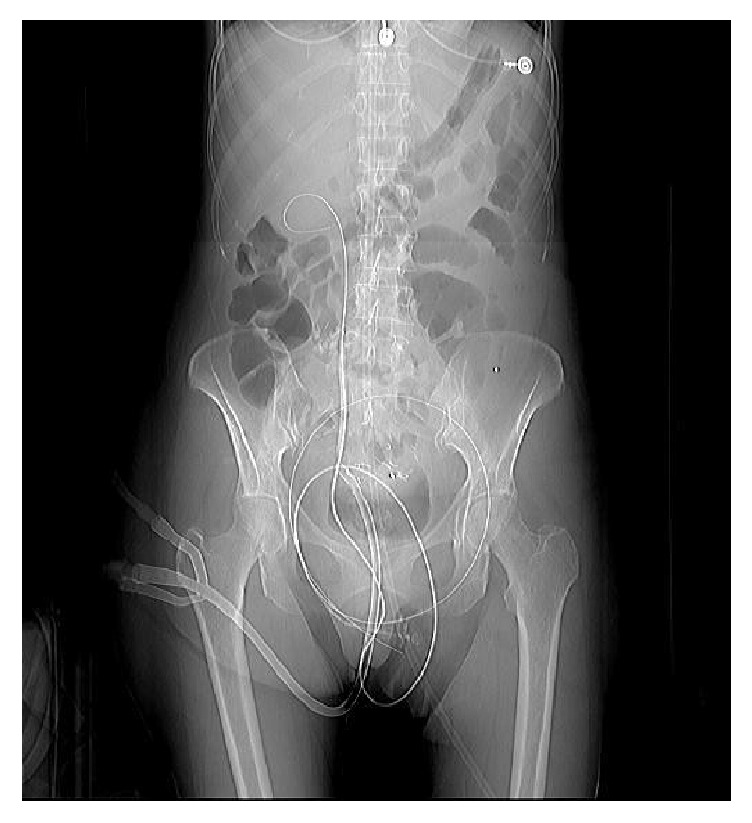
X-ray: probe with endoureteral balloon in place in the right ureter at the level of the fistula with the right primitive iliac artery.
